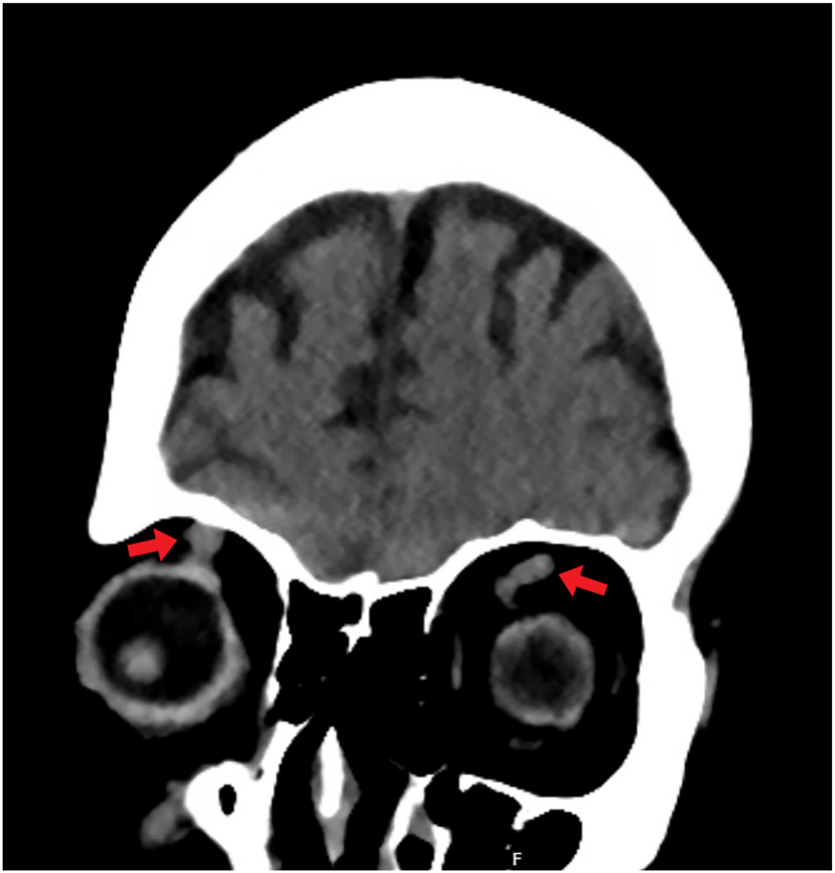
Computed tomography of the head. Coronal en face view. Enlargement of the superior ophthalmic veins is noted as a consequence of severe tricuspid regurgitation and markedly elevated venous pressure.
